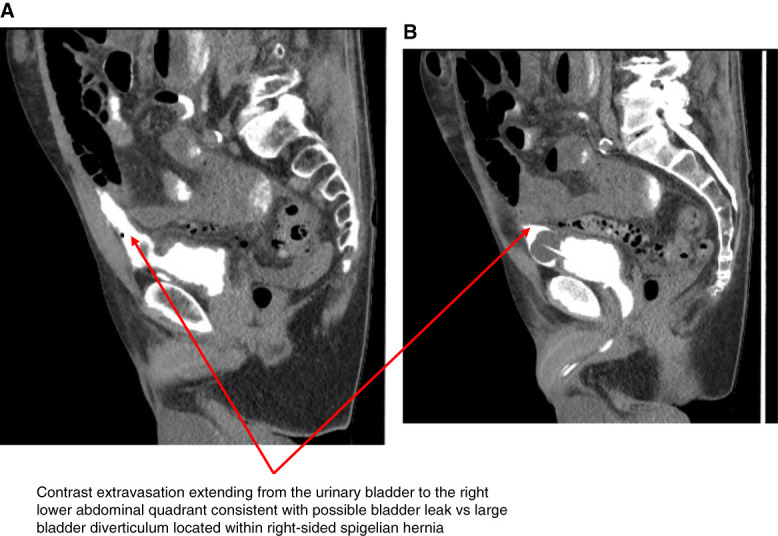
CT cystography. (A) Contrast extravasation extending from the urinary bladder to the right lower abdominal quadrant consistent with possible bladder leak versus (B) large bladder diverticulum located within right-sided spigelian hernia.
